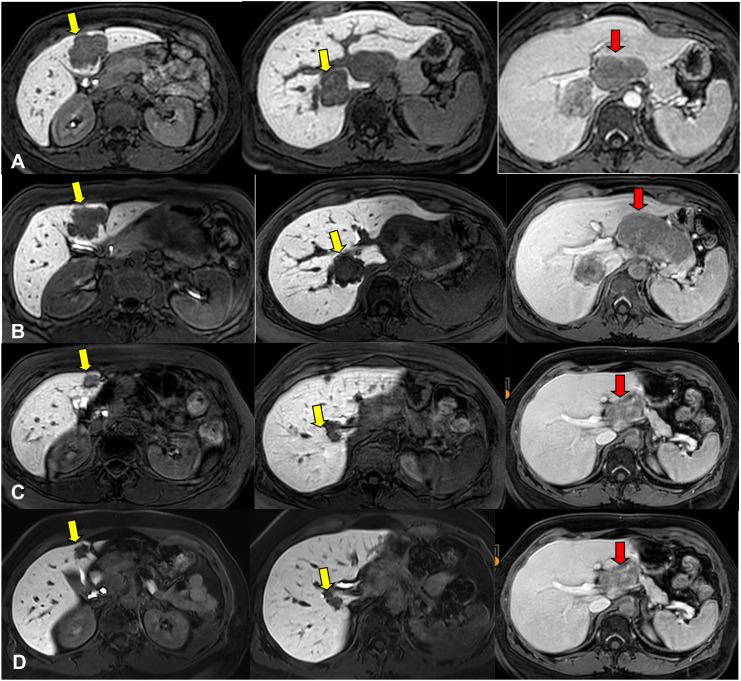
MR images acquired using a 1.5 T scanner (Philips Ingenia) showing: (A) liver lesions in segments 4B and 7 (A, B, yellow arrows) acquired during the hepatobiliary phase. Hilar adenopathy is shown in the venous T1 phase (C, red arrow). The images were obtained in November 2022, prior the initiation of systemic therapy. (B) liver lesions in segments 4B and 7 (A, B, yellow arrows) acquired during the hepatobiliary phase. Hilar adenopathy is shown in the venous T1 phase (C, red arrow). The images were obtained in April 2023, prior the initiation of radiotherapy. (C) liver lesions in segments 4B and 7 (A e B, yellow arrows) acquired during the hepatobiliary phase. Hilar adenopathy is shown in the venous T1 phase (C, red arrow). The images were obtained in March 2024, six months after the end of the radiotherapy. (D) showing liver lesions in segments 4B and 7 (A, B, yellow arrows) acquired during the hepatobiliary phase. Hilar adenopathy is shown in the venous T1 phase (C, red arrow). The images refer to the last MR evaluation in May 2025.
